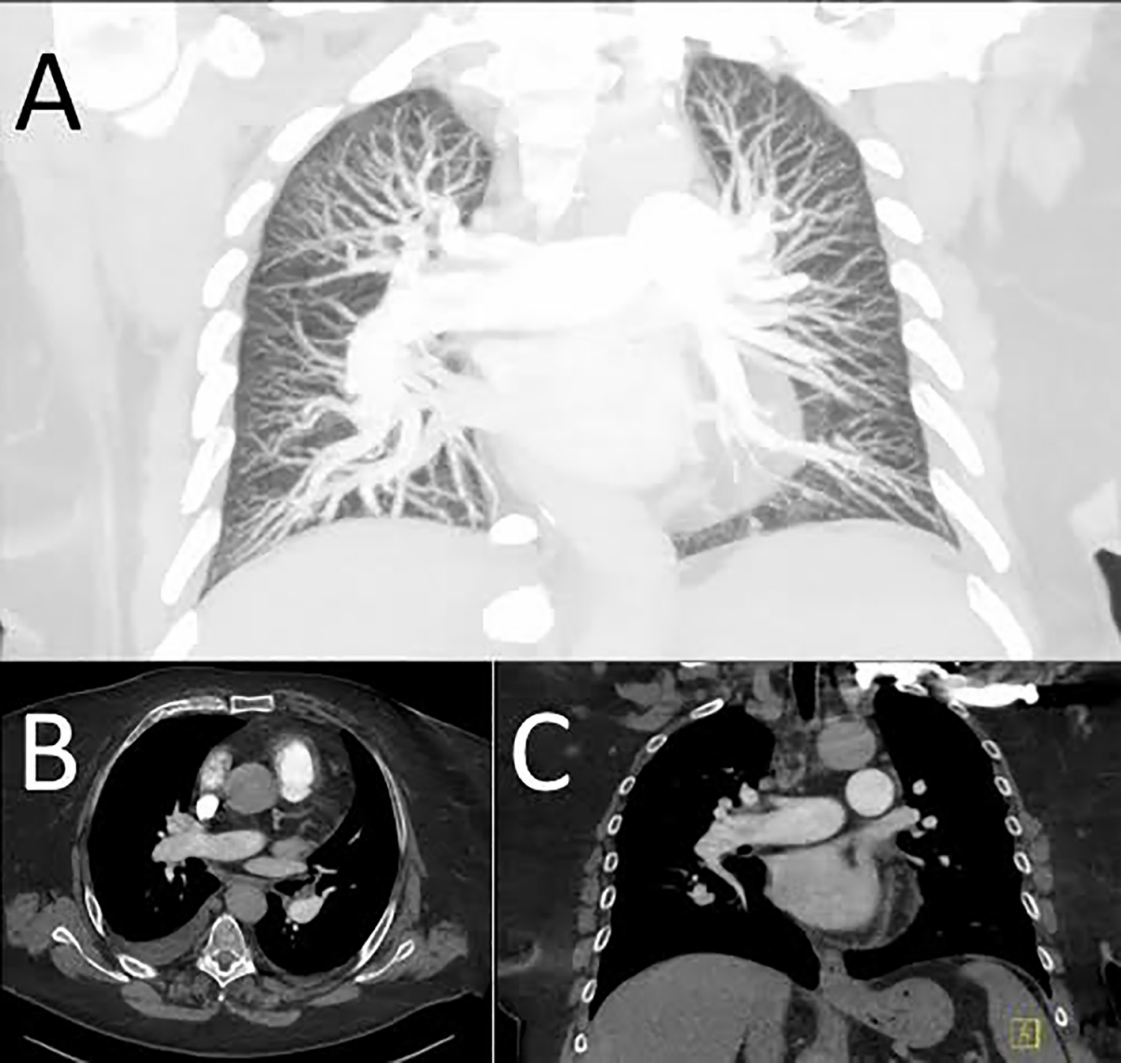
High-pitch CT pulmonary angiogram shown as coronal maximum intensity projection (A), as well as transverse (B) and coronal (C) multiplanar reformats in a 63-year old obese female patient (effective chest diameter 37.3 cm) with no evidence of pulmonary embolism. Image quality was rated as "good" on the central and lobar arteries and “sufficient” in the segmental arteries by both readers.
